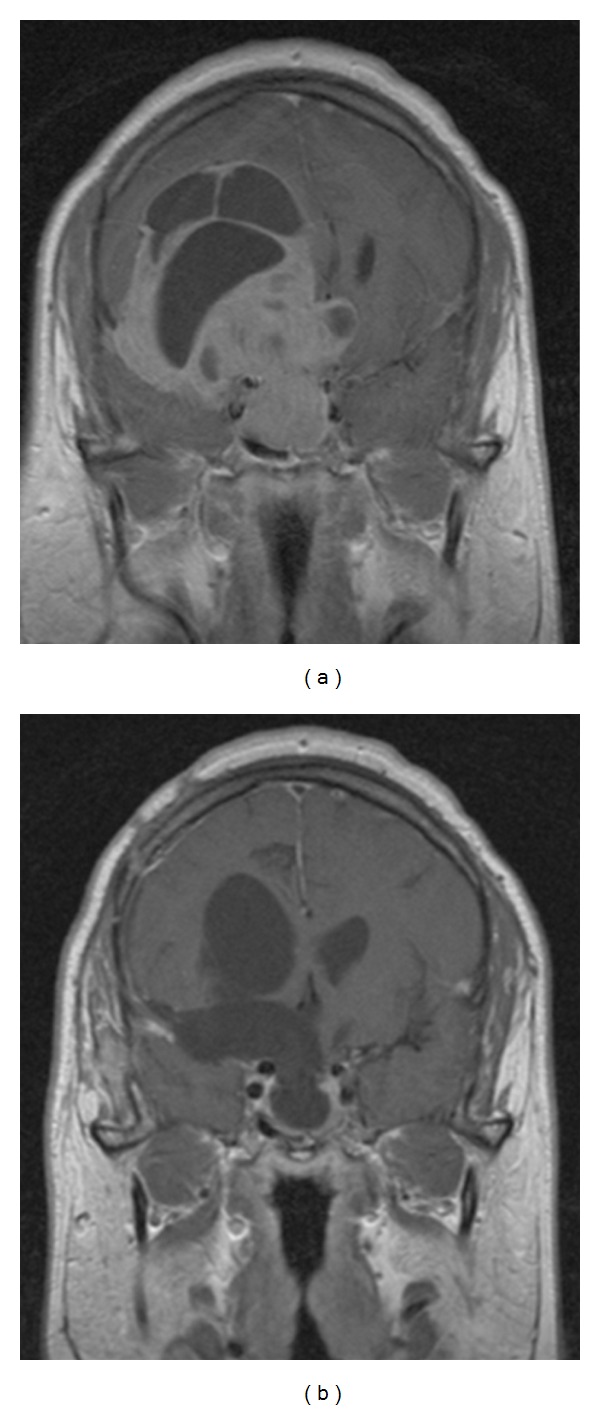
Enhanced MRI coronal view of a giant tumor growing toward the middle cranial fossa (a). A combined TCr approach was used in this case and even though the whole tumor could be removed (b), acromegaly persisted and the patient was treated with somatostatin analogues.
